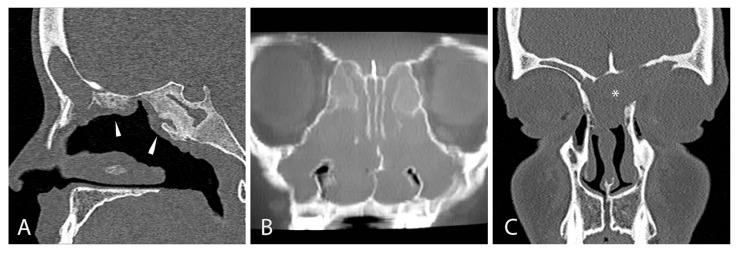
Radiological CT scan signs of chronic rhinosinusitis in 8-year-old male patients with CF: (A) sagittal CT scan view showing the presence of hyperostosis at the ethmoidal roof and sphenoidal sinus due to chronic inflammation, pointed out by white arrowheads; (B) coronal CT scan view showing bilateral massive nasal polyposis; (C) coronal CT scan view showing left fronto-ethmoidal empyema (*). Parent/guardian consent to use clinical pictures of the patients was obtained.
